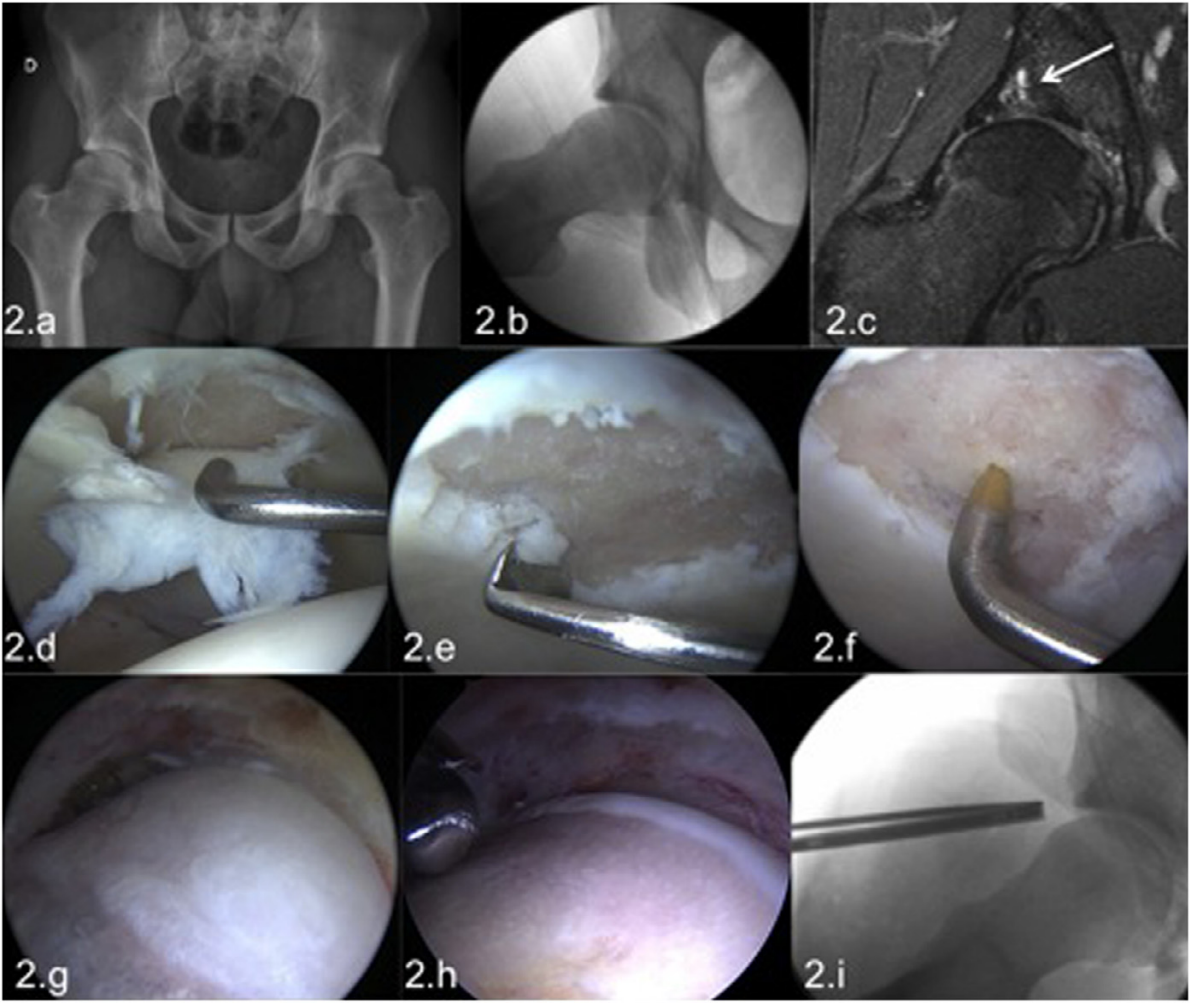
Male patient, 23 years old, medical student and amateur rugby player with sports-related pain in the right hip. The radiology demonstrated a FAI with a significant CAM deformity without significant joint space narrowing (2a, 2b). MRI showed a labral injury, a focal chondral lesion with subchondral cysts, and subchondral edema (2c). In spite of these ominous imaging signs given his young age and no other signs of osteoarthritis, hip arthroscopy was performed. At the arthroscopy an extensive full-thickness chondral lesion was found in the load-bearing surface of the acetabulum (2d); no femoral head cartilage lesions were present. The chondral lesion was treated by abrasive chondroplasty (2e) and microfractures (2f). In the peripheral compartment the extensive femoral bump (2g) was resected (2h). Intraoperative dynamic testing at the end of the femoroplasty demonstrated absence of impingement; the axial radiography demonstrated a satisfactory correction of the cam deformity (2i). Nevertheless after this arthroscopic repair, the future of this joint is uncertain with a high risk to progress to osteoarthritis in this young patient.
